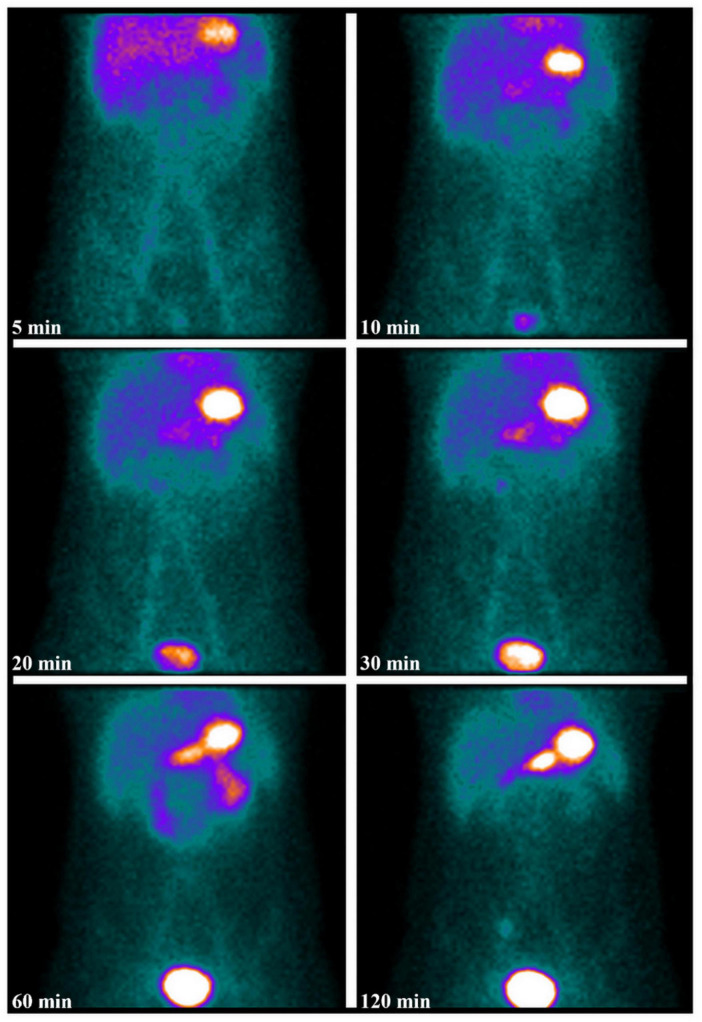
A 10-year-old boy presented with intermittent rectal bleeding and melena, with a hemoglobin level of 71 g/L. Serial [^99*m*^Tc]pertechnetate scintigraphy images obtained within 60 min showed no significant abnormal radiotracer accumulation in the abdomen or pelvis; however, delayed imaging at 120 min revealed abnormal radiotracer uptake in the right lower quadrant. The diagnosis of MD was confirmed postoperatively. A review of the patient’s history revealed that he had undergone gastrointestinal bleeding scintigraphy (*in vivo* red blood cell labeling scan) at an outside hospital 1 day prior, which may have influenced the results of the [^99*m*^Tc]pertechnetate scintigraphy.
